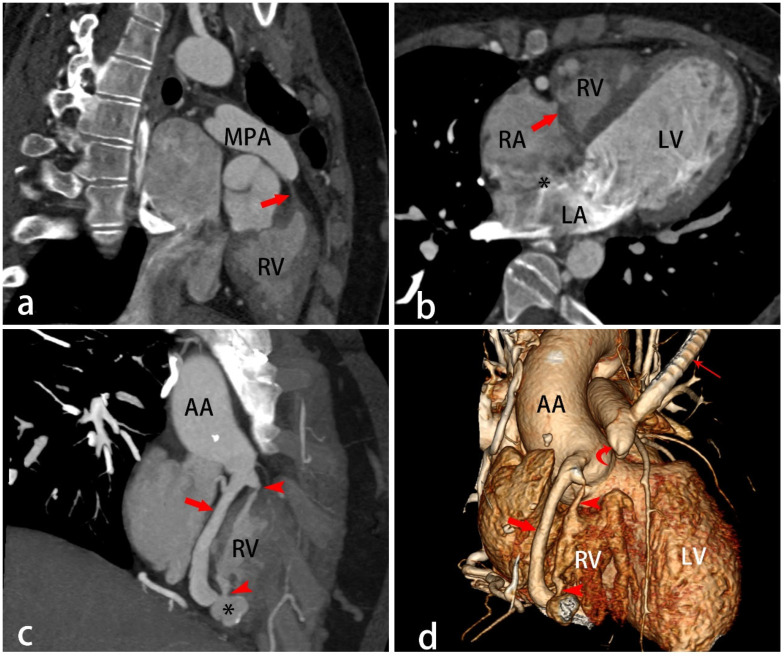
A 17-year-old female with pulmonary atresia with intact ventricular septum. CT angiography oblique sagittal view shows atresia of the right ventricular outflow tract ((a), red arrow). Four-chamber view reveals tricuspid atresia ((b), red arrow), atrial septal defect ((b), black asterisk), and intact ventricular septum. The coronary artery is dilated ((c,d), red arrow) with aneurysm formation ((c), black asterisk), and right ventricle–coronary artery fistulas are present ((c,d), red arrowheads). CT three-dimensional volume-rendered images demonstrate no connection between the right ventricle and the main pulmonary artery ((d), red curved arrow). A Blalock–Taussig (B–T) shunt was performed to improve pulmonary blood flow ((d), thin red arrow). LV, left ventricle; LA, left atrium; RV, right ventricle; RA, right atrium; AA, ascending aorta; MPA, main pulmonary artery.
